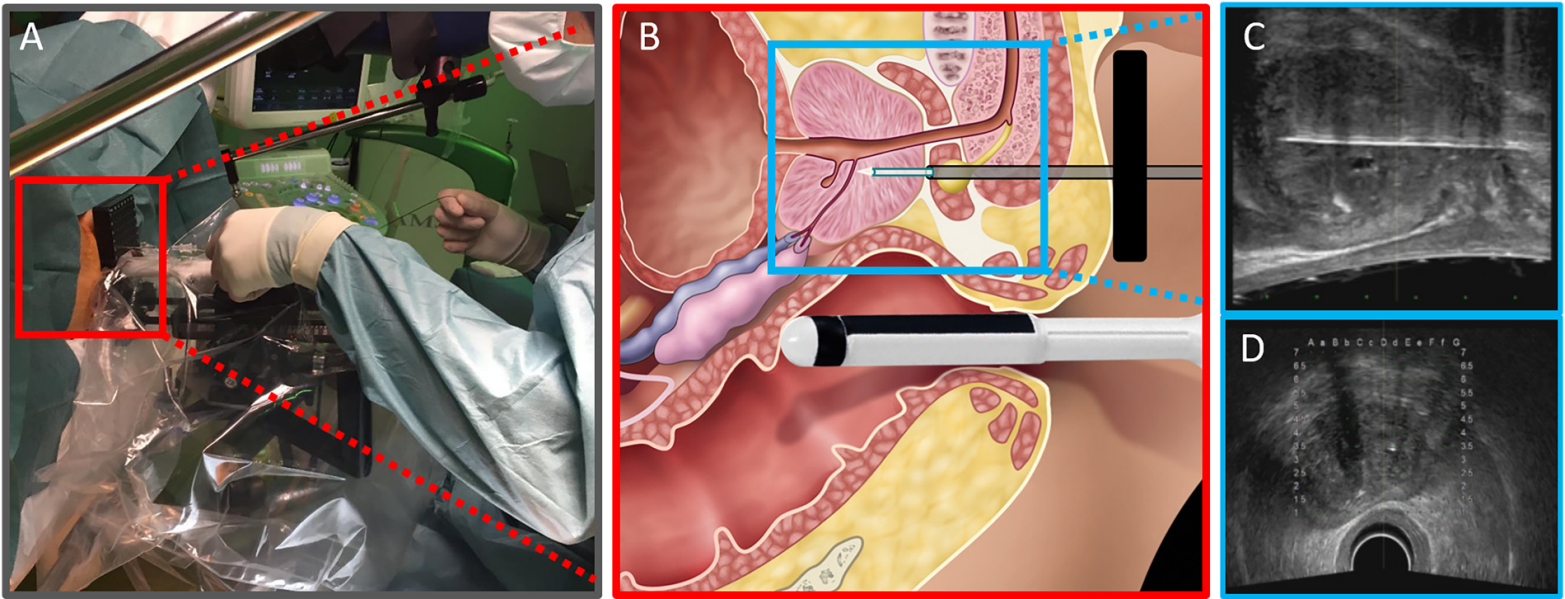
(A) An overview of a TTMB setting in the operating room including (B) a CLE measurement of the prostate with a forward imaging Cellvizio® AG-Flex 19 fiber optic mini probe-based system under transrectal ultrasound guidance using (C) sagittal and (D) axial planes. It was adapted from “The First In Vivo Needle-Based Optical Coherence Tomography in Human Prostate: A Safety and Feasibility Study” by Swaan *et al*, 2019, Lasers in surgery and medicine.
